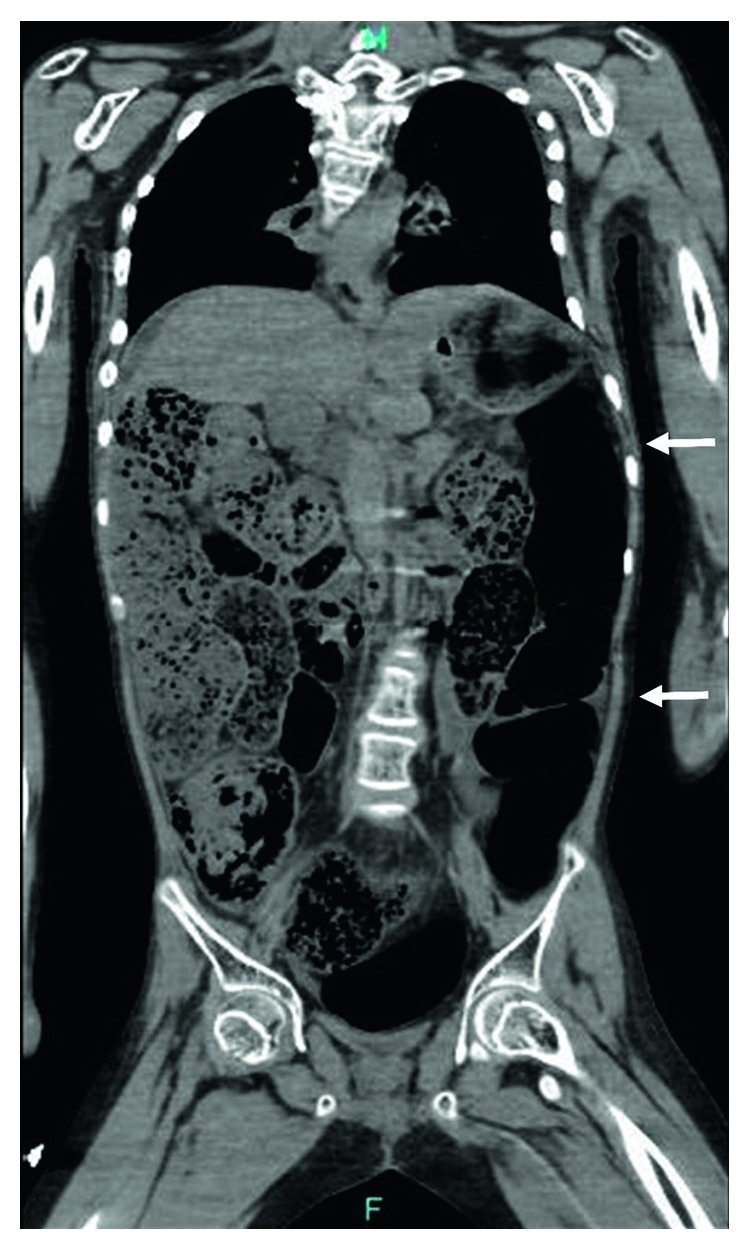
Abdominal computed tomographic image. The arrows show the patient's dilated and thinned intestines from the duodenum to the sigmoid colon.
